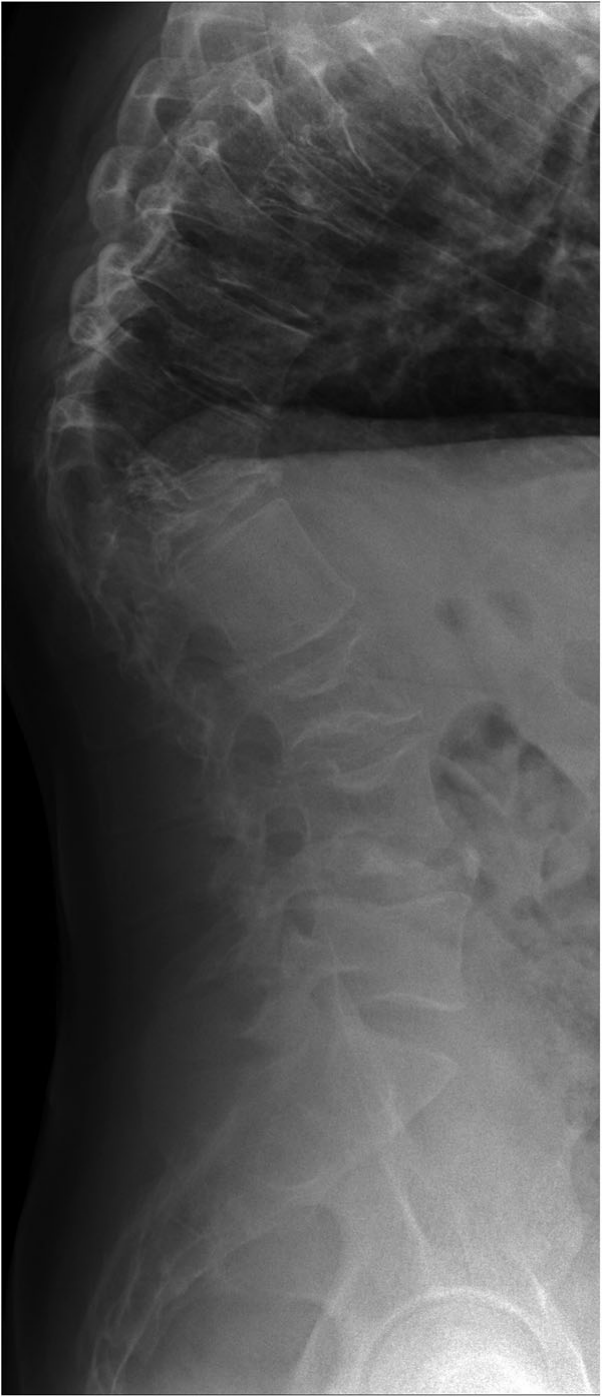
Lateral radiograph of the spine of a patient with type 3 GD, showing an abruptly angulated thoracic kyphosis
